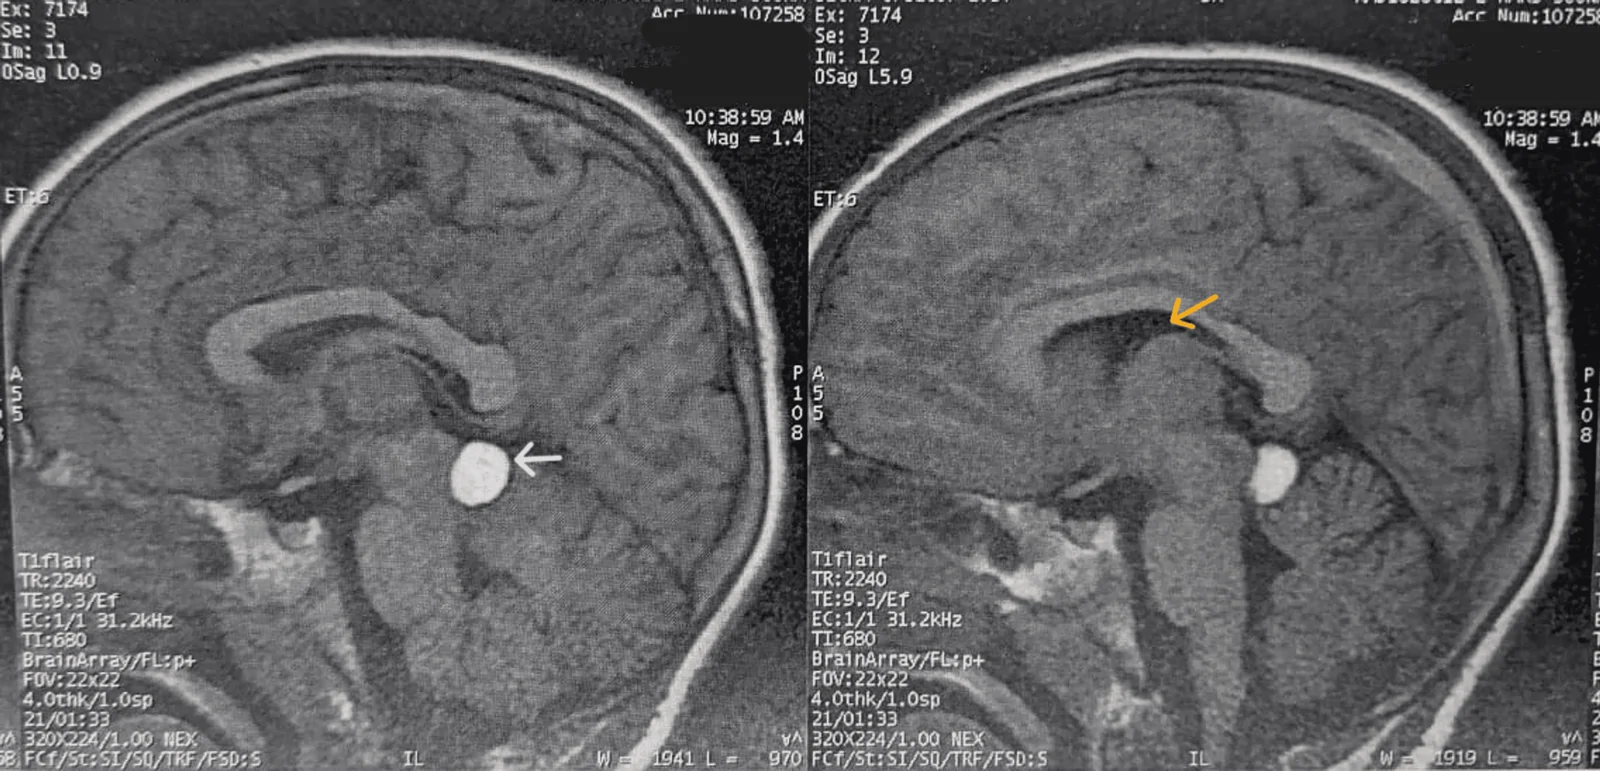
Sagittal fluid-attenuated inversion recovery images magnetic resonance imaging brain image showing lesion in the quadrigeminal cistern (white arrow) without upstream hydrocephalus (yellow arrow)
